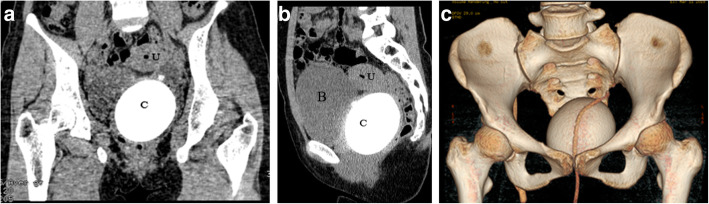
The multiple planar reconstruction (MPR) (a, b) and volume rendering (VR) (c) of pelvic CT, showing a large calculus in the vagina. B, bladder; U, uterus; C, calculus
